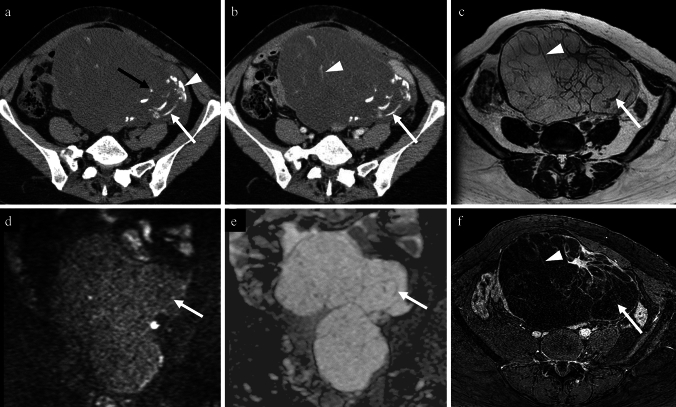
49-year-old woman with a mucinous cystadenoma of the left ovary. a non-contrast CT; b contrast-enhanced CT; c axial T2-weighted image; d coronal diffusion-weighted image; e coronal apparent diffusion coefficient map; f axial contrast-enhanced T1-weighted image. A cystic tumour containing multiple septa is observed, with predominant linear calcifications aligned with the septa (a; white arrow), accompanied by areas of punctate (a; black arrow) and coarse calcifications (a; white arrowhead) on non-contrast-CT. Contrast-enhanced CT revealed enhancement along the septa (b, arrowhead), however the enhancement effect of the calcified septa is unclear (b, arrow). On T2-weighted image, the cystic content appears relatively homogeneous, and the calcified septa (c; arrow) is slightly thicker than the non-calcified ones (c; arrowhead). No diffusion restriction is noted on the diffusion-weighted image (d and e; arrows). The post-contrast T1-weighted image shows smooth enhancement along the septa (f; arrowhead), but the enhancement effect of the calcified septa is not evident (f; arrow). Pathological examination revealed a mucinous cystadenoma
